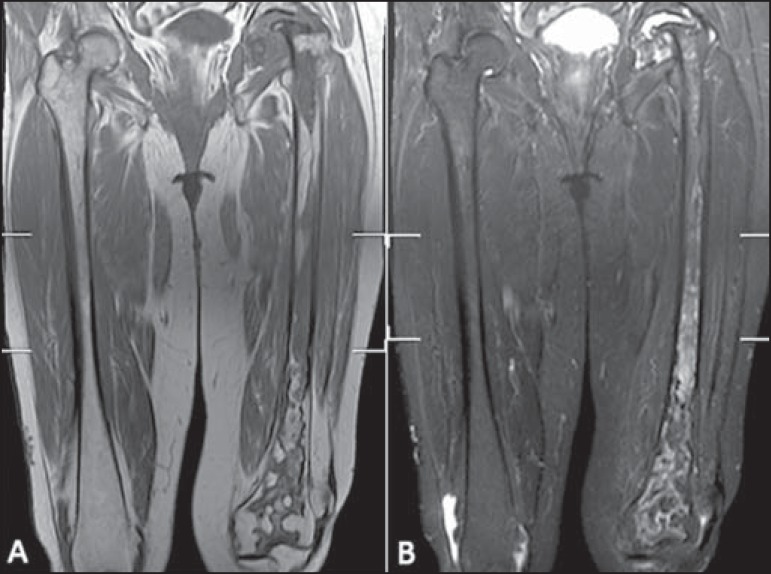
MRI images of a 63-year-old woman with Gaucher’s disease. Coronal T1-weighted
